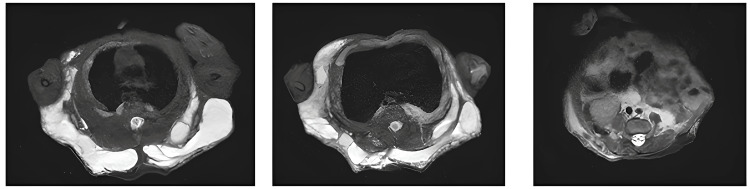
Day 10 post-natal MRI centered on the lymphatic malformation Axial T2 fat sat views of the macrocystic lymphatic malformation of the posterior wall with subcutaneous, muscular, and profound involvement (left pleural, retroperitoneal).
